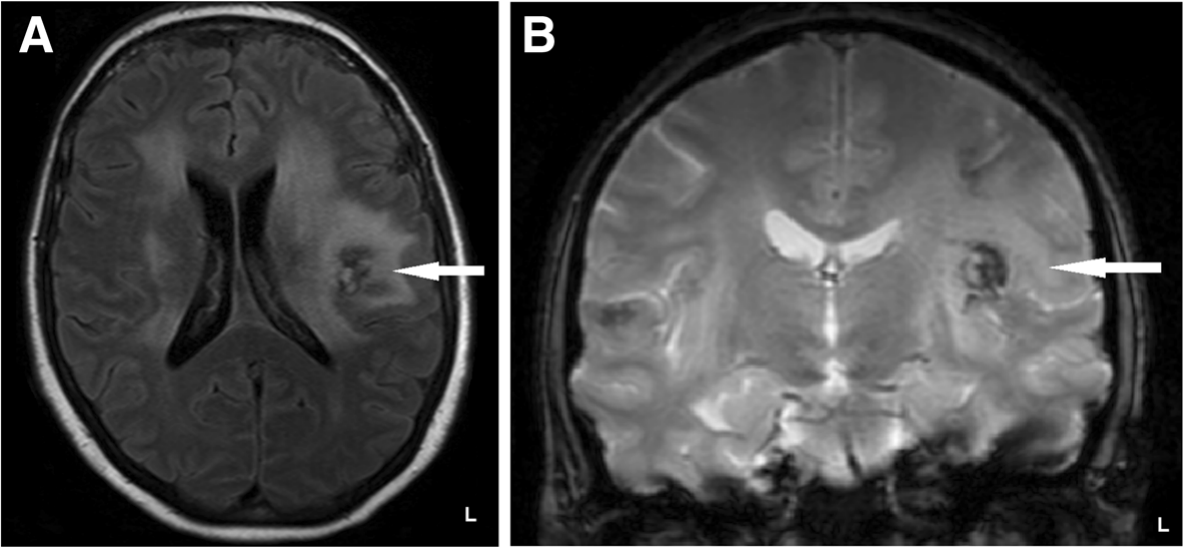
Cranial MRI images of the second inflammatory episode. a. Axial T2 fluid attenuation inversion recovery (FLAIR)-weighted MRI indicating large, high-intensity periventricular lesions, and a low-intensity left frontoparietal lesion. b. Coronal T2*-weighted gradient-echo MRI confirming the presence of a low-intensity lesion, suggestive of hemosiderin deposits, that had not been found on previous MRIs
